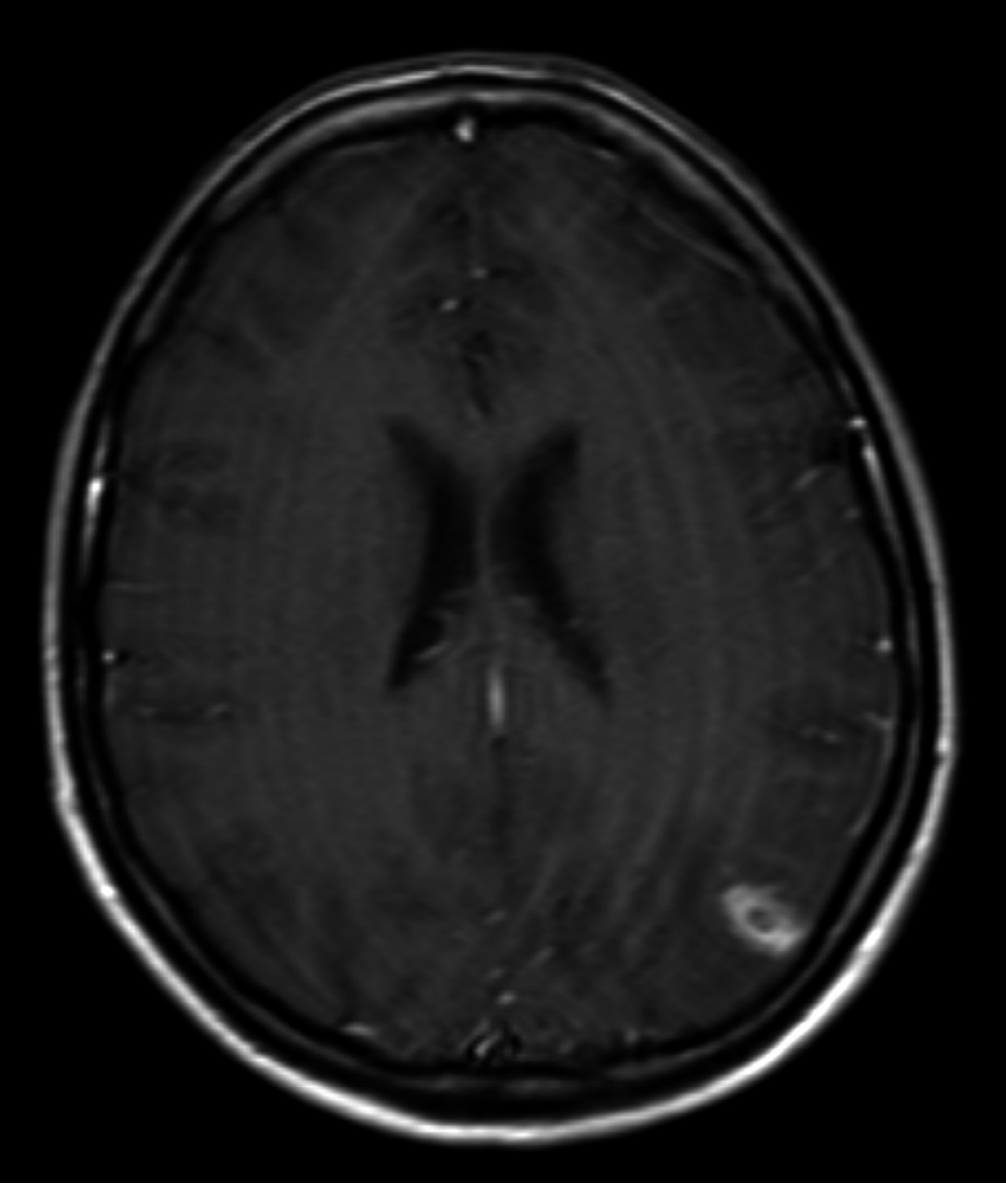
MRI with contrast demonstrating ring‐enhancing lesion in the Left Posterior watershed territory.
